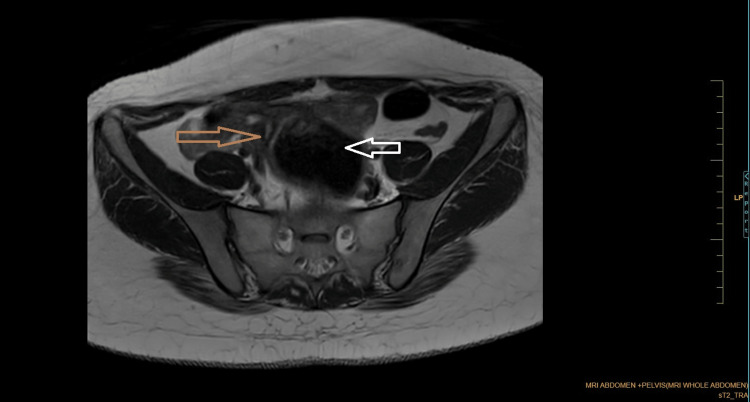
Axial T2WI showing two widely separated divergent uterine horns with fundal cleft. There is evidence of cystic collection appearing hypointense on T2 communicating with the left-sided cervix and uterus (white arrow). On the right side, normal uterus and cervix are present but compressed and pushed laterally by hematocolpos (brown arrow). Note: Authors' original image T2WI: T2-weighted image
